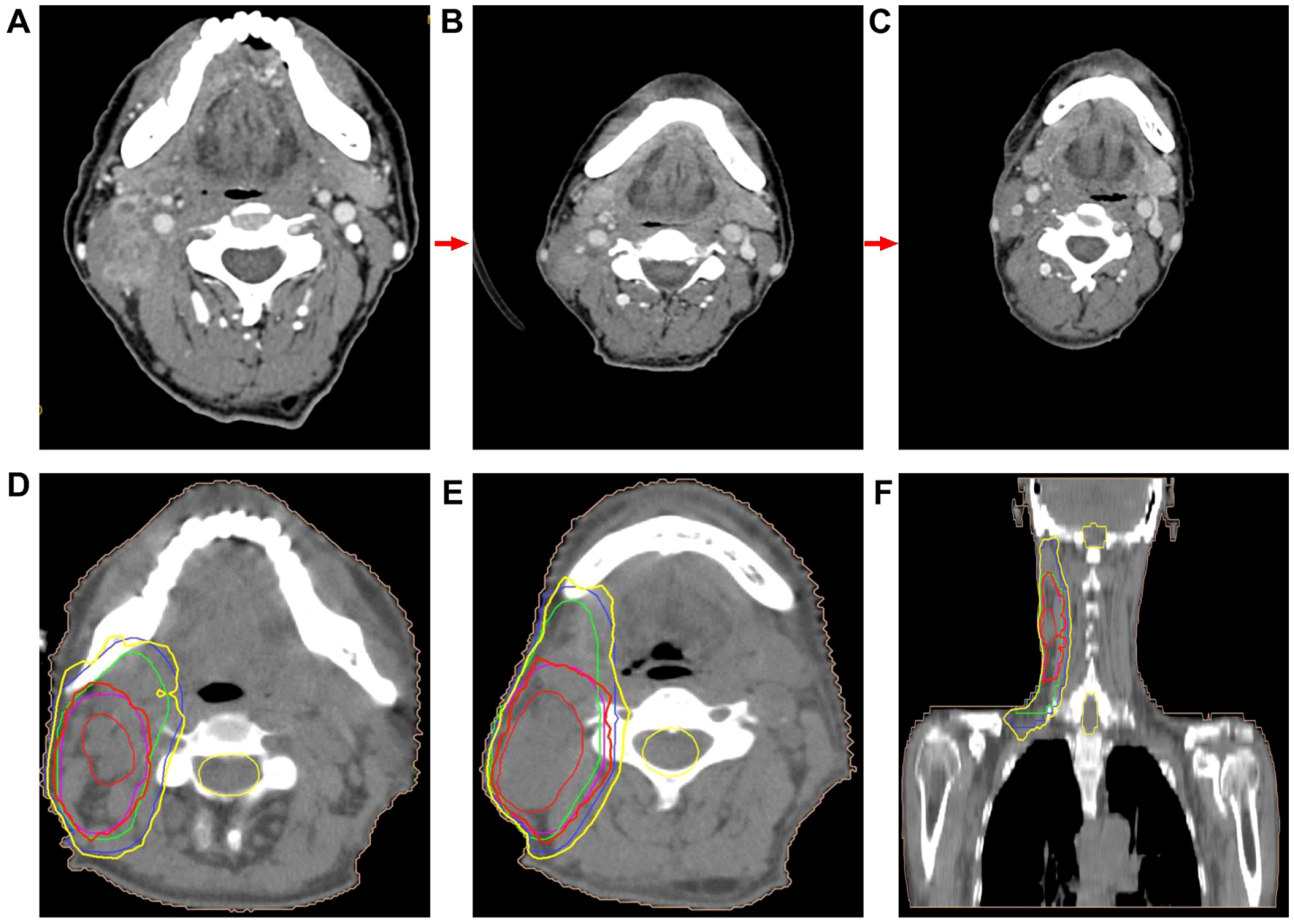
(A) CT image demonstrated cervical metastatic lymph nodes (on May 29, 2023). (B) CT image showed that cervical metastatic lymph nodes achieved partial remission about two months after the initial camrelizumab administration (on August 17, 2023). CT image revealed that metastatic lymph nodes in the neck achieved partial remission were further reduced nearly 4 months after the end of radiotherapy (follow-up on February 26, 2024). (D–F) Radiation field.
